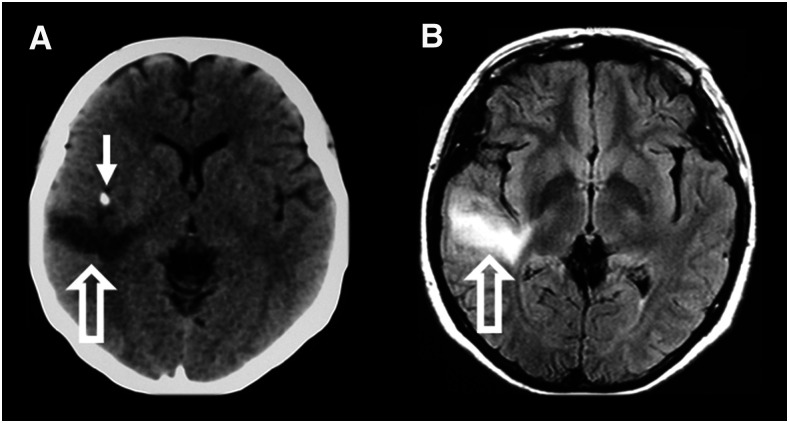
Inflamed calcifications. CT scan (A) and concurrent T2-FLAIR MRI scan (B) demonstrating axial views of a calcified lesion with perilesional edema seen in the right temporal lobe of both the CT scan and the MRI scan. The calcified lesion is indicated by a solid arrow. Areas of perilesional edema are indicated by hollow arrows.
